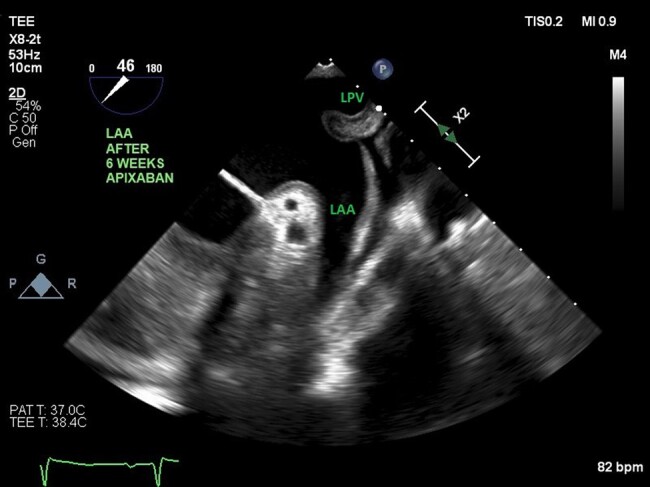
Resolution of left atrial appendage thrombus on apixaban therapy for 6 weeks (transoesophageal echocardiogram of left atrial appendage at 46°). LPV, left pulmonary vein.
